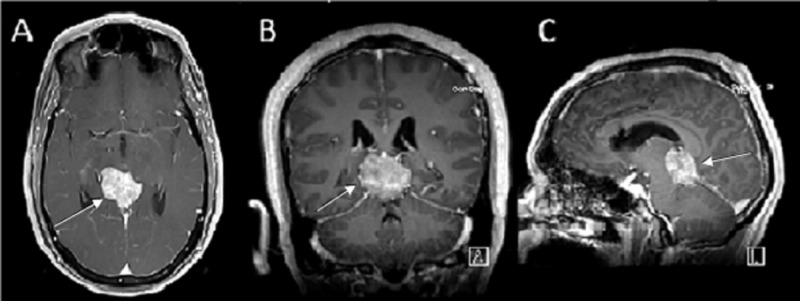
Gadolinium enhanced T1-weighted pre-operative magnetic resonance imaging (MRI) with (A) axial, (B) coronal and (C) sagittal view showing a homogenously enhancing lesion, the region of the pineal gland.
